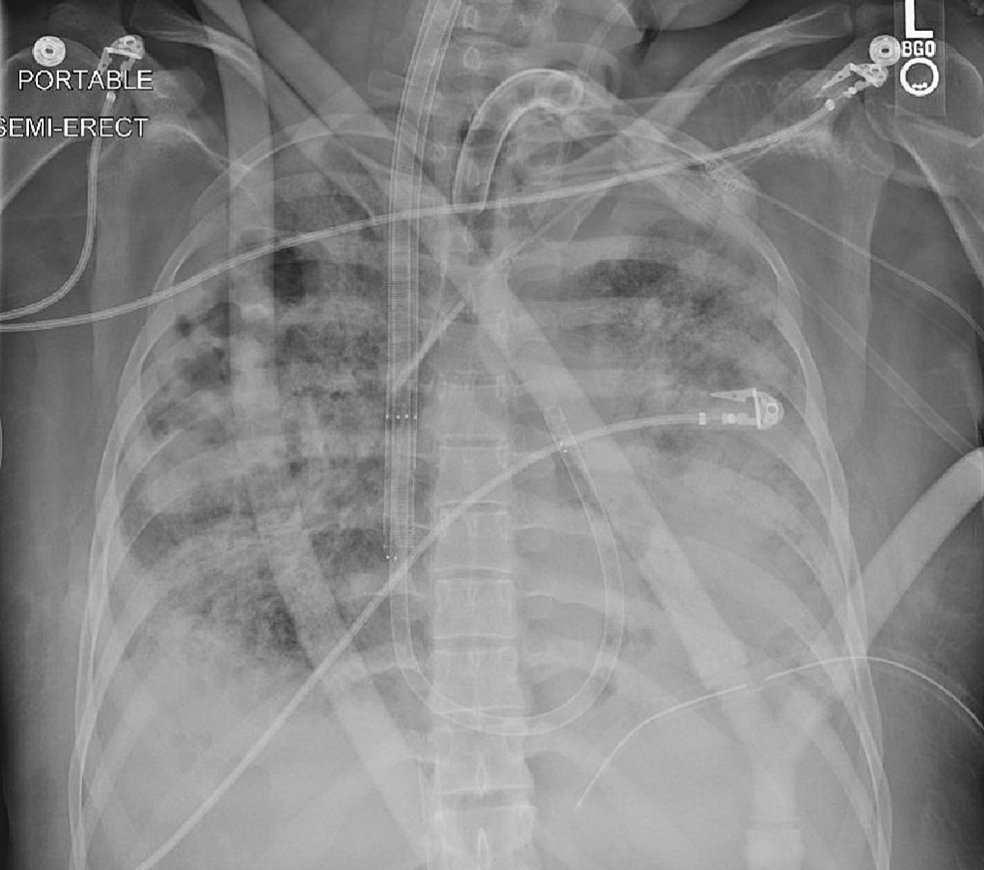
Interval Radiograph One-Month Post-admission Significant diffuse airspace disease is still apparent.
